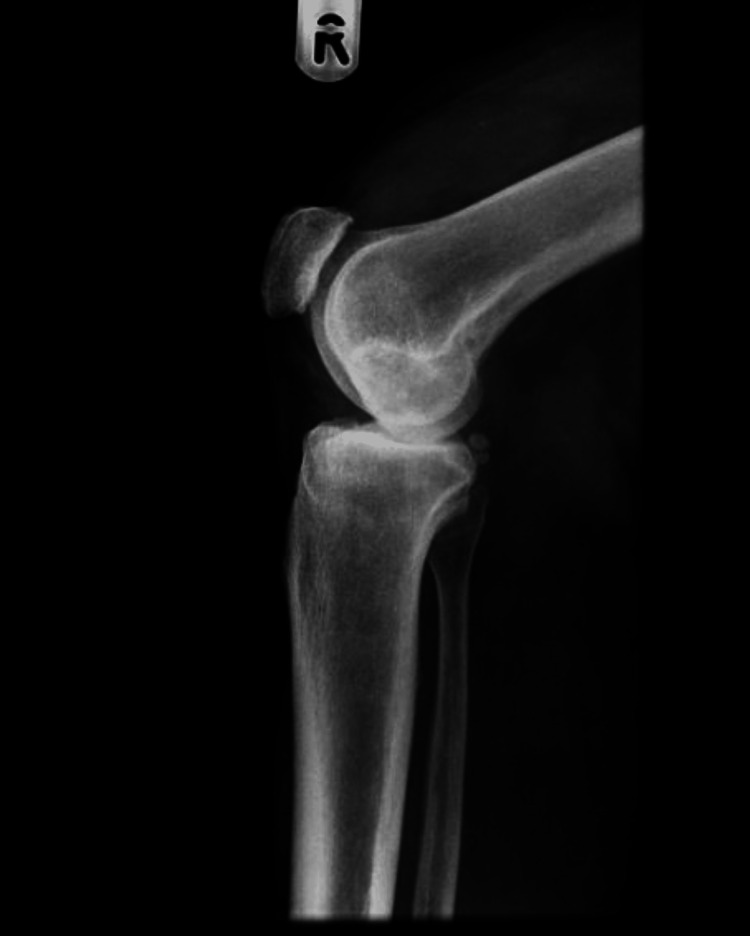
Preoperative X-ray, lateral view
